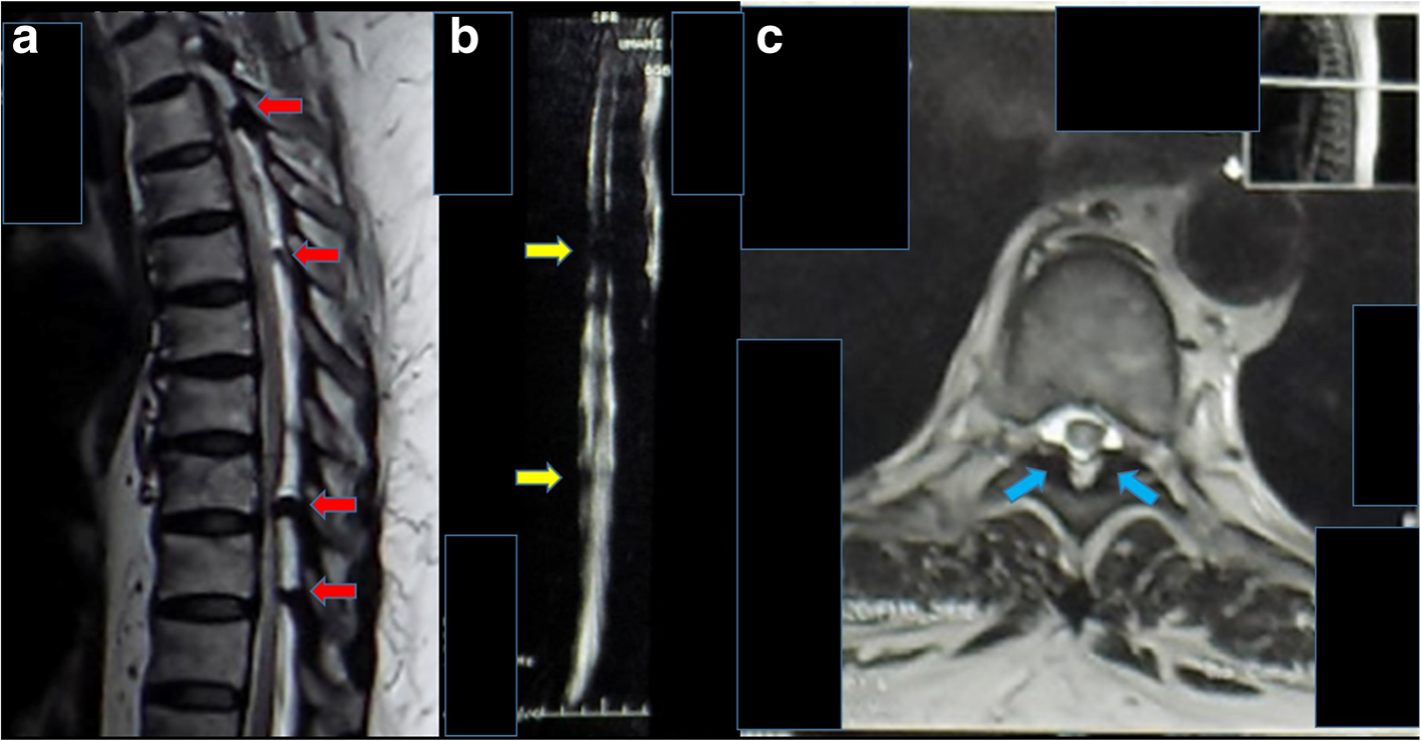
Sagittal and axial views of thoracic magnetic resonance imaging with T1-weighted and T2-weighted images. a Sagittal section of T2-weighted magnetic resonance imaging shows many compressions of spinal cord at levels T3, T5, T8, and T9 (*red arrows*). b Magnetic resonance imaging-myelography that shows spinal cord compression at levels T5 and T9 (*yellow arrows*). c Axial view of T5 vertebrae level shows the hypointense mass at left and right posterior parts (*blue arrows*). The mass compresses the canal
